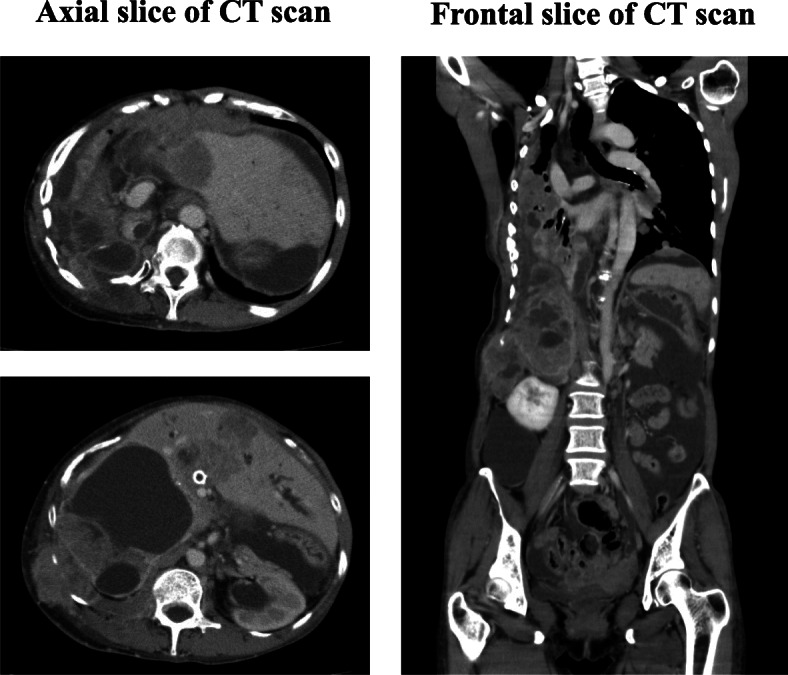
Diagnostic computed tomography (CT) image before the patient’s death. Axial slice and frontal slice CT scans at 4 weeks before the patient’s death. The tumor invaded into the subcutaneous tissue via the intercostal muscle. Furthermore, the tumor invaded into the pleural cavity from the liver surface via the right diaphragm
